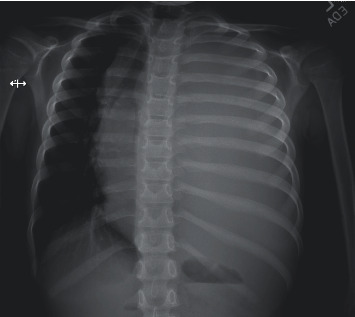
Chest radiograph with complete left-sided pleural effusion with a resultant mass effect and significant deviation of the mediastinum to the right.
